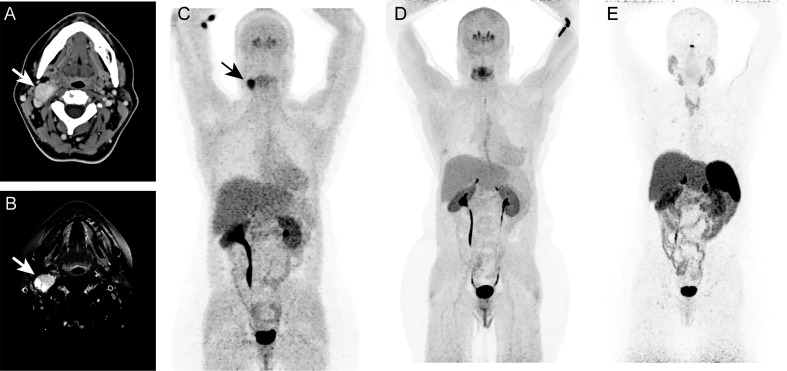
In this figure of a 47-year-old-woman, axial images (A, B) of contrast enhanced computed tomography (CT, A) and T1 weighted, fat suppressed magnetic resonance imaging (B) demonstrates an intensely enhancing 2.7 x 2.0 cm mass (arrows; A, B) at the bifurcation of right common carotid artery causing splaying of right internal and external carotid arteries. The anterior maximum intensity projection (MIP, C–E) of ^18^F-fluorodopa (^18^F-FDOPA) positron emission tomography (PET) demonstrates an uptake in the carotid region (arrow, C). This mass was identified as a right carotid body paraganglioma. Six years later, follow-up functional PET/CT imaging with ^18^F-FDOPA (D) and ^68^Ga-DOTATATE (E) shows no evidence of pheochromocytoma/paragangliomas (PHEOs/PGLs). Similarly, whole-body CT (not shown here) imaging and neck MRI (not shown here) were negative for PHEOs/PGLs.
